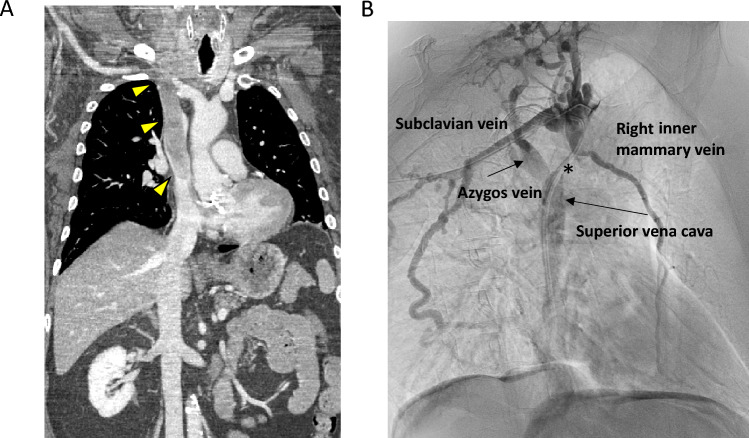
A Extensive thrombus from the right jugular vein to the right atrium (yellow triangles) of patient #1 at CT-scan. B Superior vena cava obstruction due to the presence of thyroid metastasis (asterisk) of patient #2 at superior vein cavography
